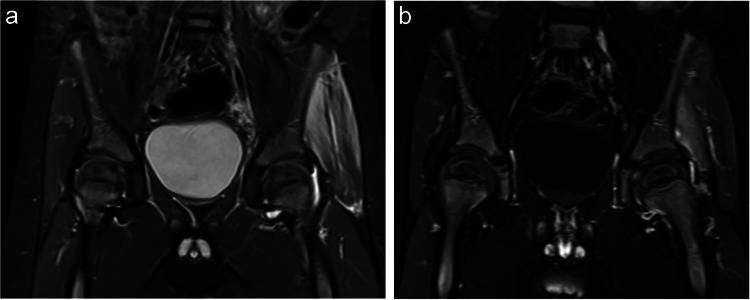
A 3-year-old boy with septic hip arthritis and myositis. The patient presented with a 3-day history of fever (38.9 °C) and refusal to bear weight. He had a normal white blood cell (WBC) count, an erythrocyte sedimentation rate (ESR) of 67 mm/h, and a C-reactive protein (CRP) level of 15 mg/dL. Left hip aspiration revealed a WBC count of 11,539 cells/mL, with a positive culture for *Staphylococcus aureus*. A coronal MRI STIR shows moderate left hip effusion and marked edema in the left gluteal muscles. B coronal MRI SET1 with fat suppression post-contrast shows normal enhancement of the left femoral head and enhancement of the left gluteal muscles
